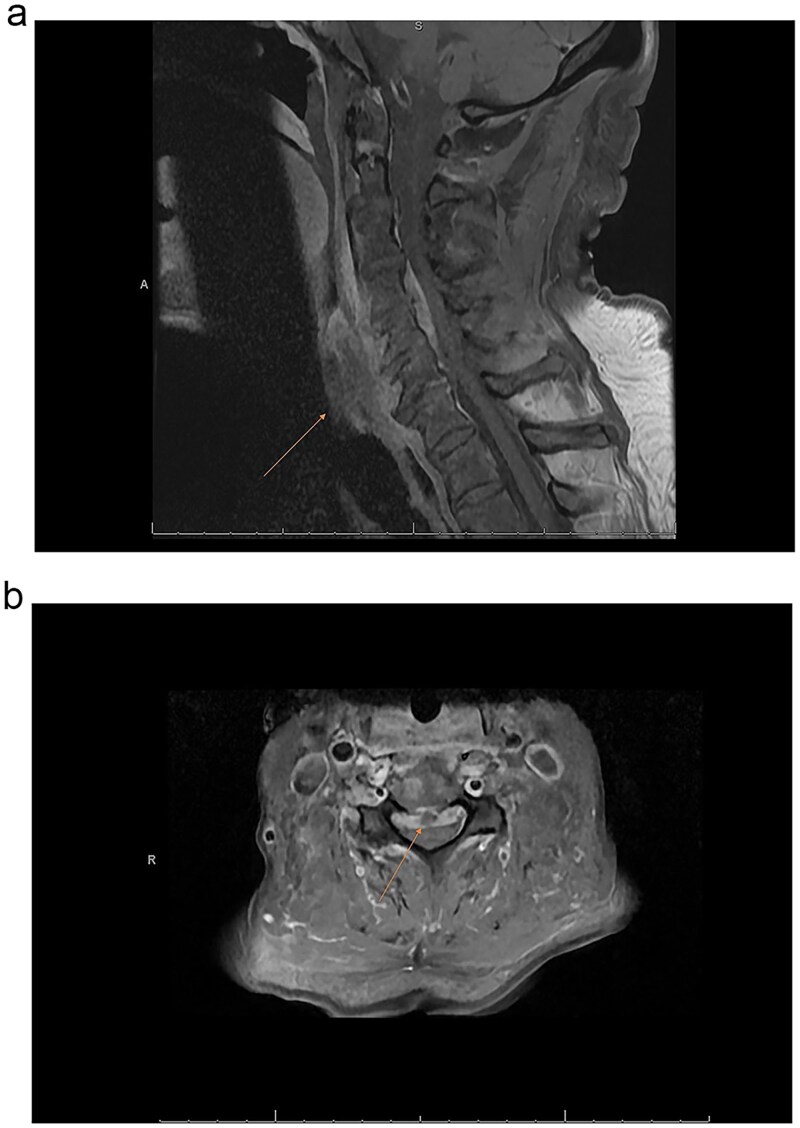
(a) MRI cervical sagittal of T1 vertebrae with gadolinium contrast depicting osteomyelitis/discitis with epidural abscess. (b) MRI cervical axial T1 image with gadolinium contrast C4–5 demonstrating epidural abscess and cord compression.
